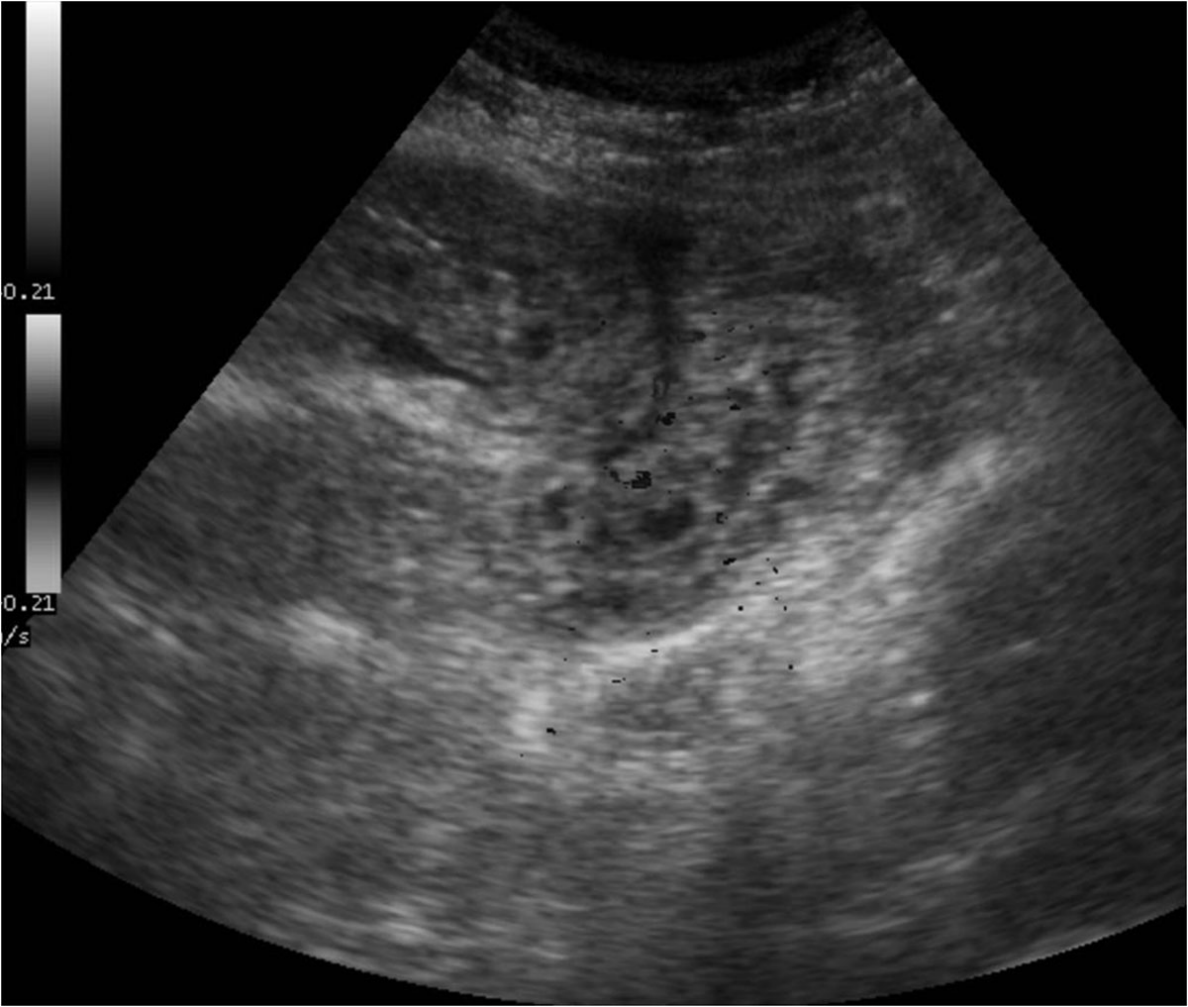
Intraperitoneal free fluid and/or reduced intestinal peristalsis at sonographic examination are considered indirect signs of gastroduodenal perforation
